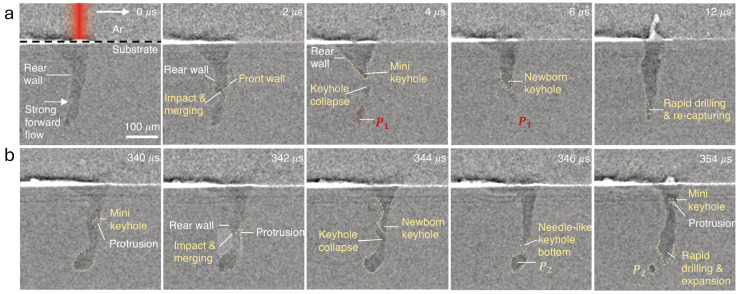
Megahertz X-ray images capturing (a) intrinsic keyhole oscillation without pore formation and (b) perturbative keyhole oscillation with keyhole pore formation [45]. The dashed lines highlight the keyhole.
